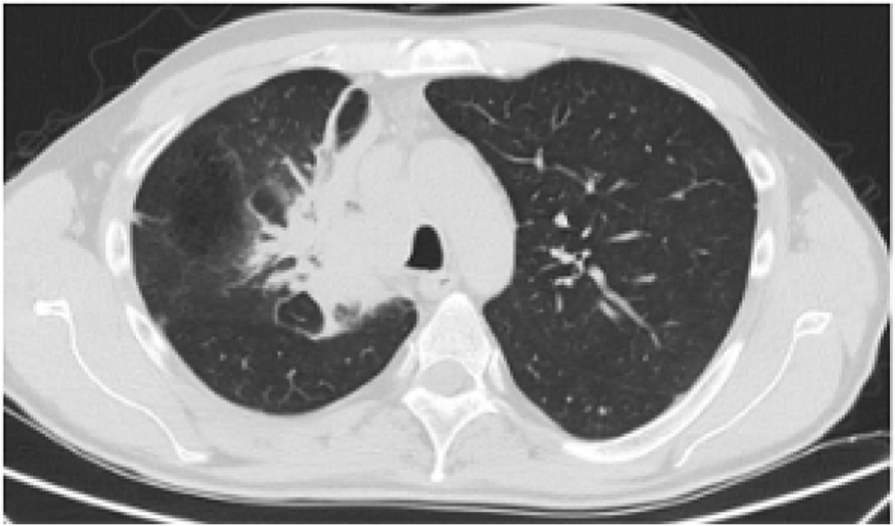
Contrast-enhanced thorax CT-scan revealing a large, enhancing, softtissue tumor of the right hemithorax
